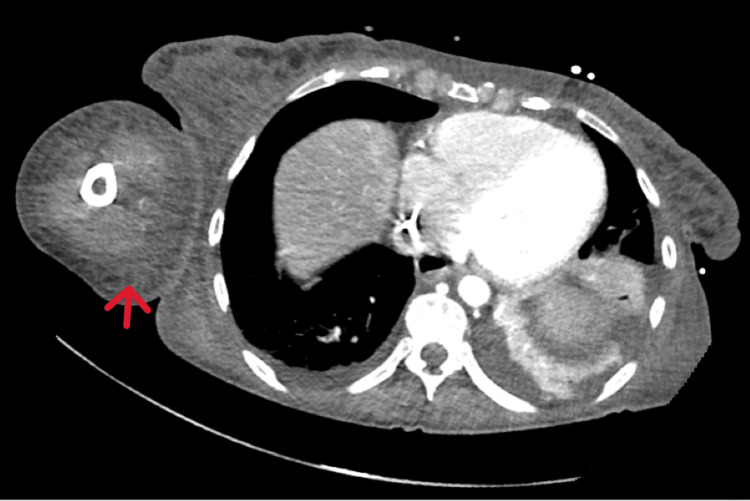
Contrast-enhanced CT scan showing thickening of the skin and subcutaneous tissue of the right arm and forearm of nonspecific appearance.
